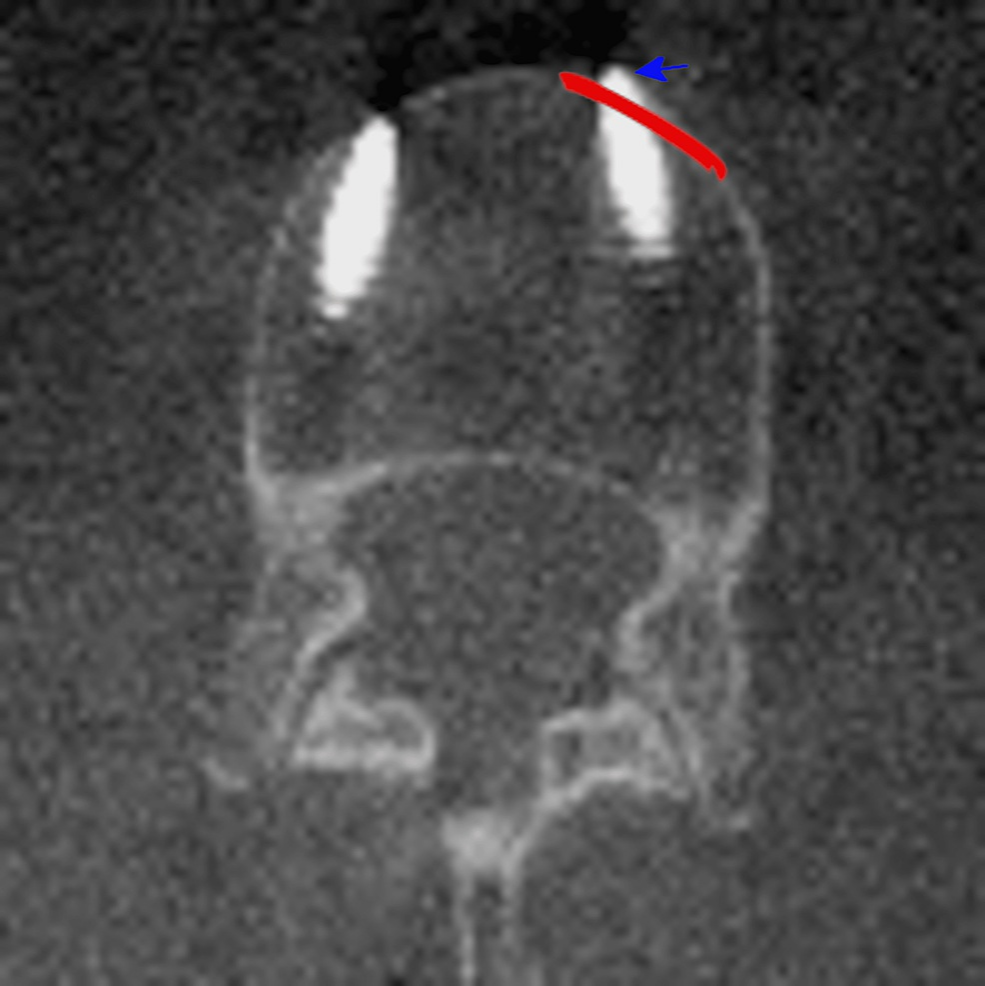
Axial CT radiograph of the left L2 anterior cortex breach on patient 4. An axial CT radiograph of the anterior cortex breach of the left L2 screw on patient 4. The anterior cortex is lined in red. A blue area denotes the screw deviation.
